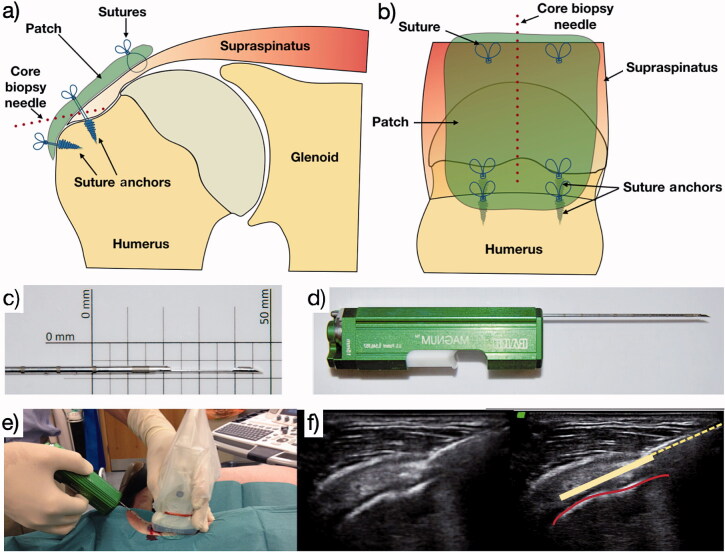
a and b: The needle site used at the 4-week postoperative biopsy; c and d: the core biopsy needle and automated firing device used; e and f: the technique for ultrasound-guided core biopsy. Red line denotes supraspinatus bony footprint. Dotted yellow line denotes core biopsy needle; solid yellow line denotes trajectory of core biopsy needle within supraspinatus tendon when deployed.
